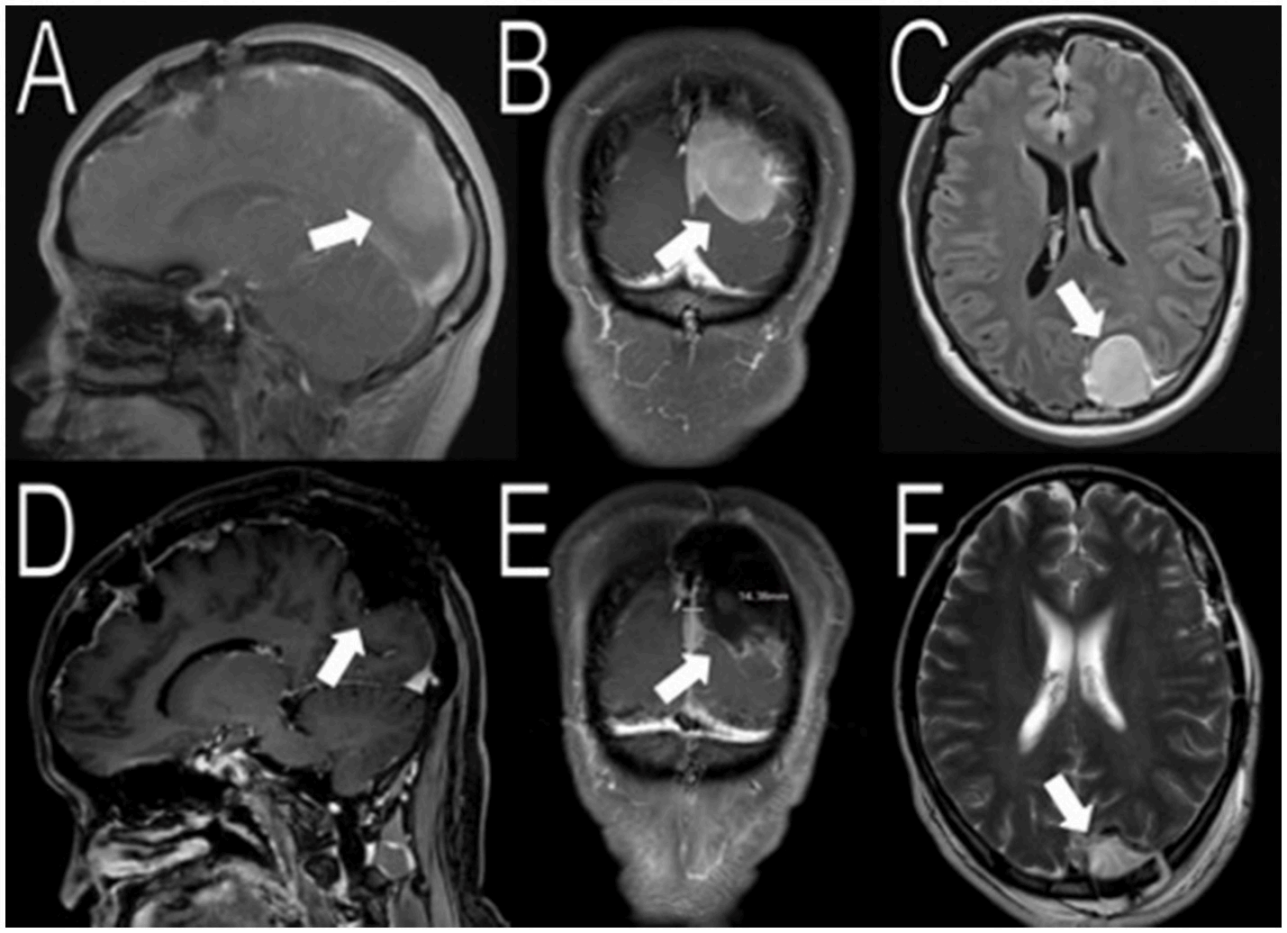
Resection of the occipital mass. Preoperative MRI showed a 2.6 × 2.9 × 3.9 cm (AP, TV, CC) mass in the left occipital convexity (white arrows in A–C). Immediate post-operative MRI showed removal of the mass (white arrows in D–F).
